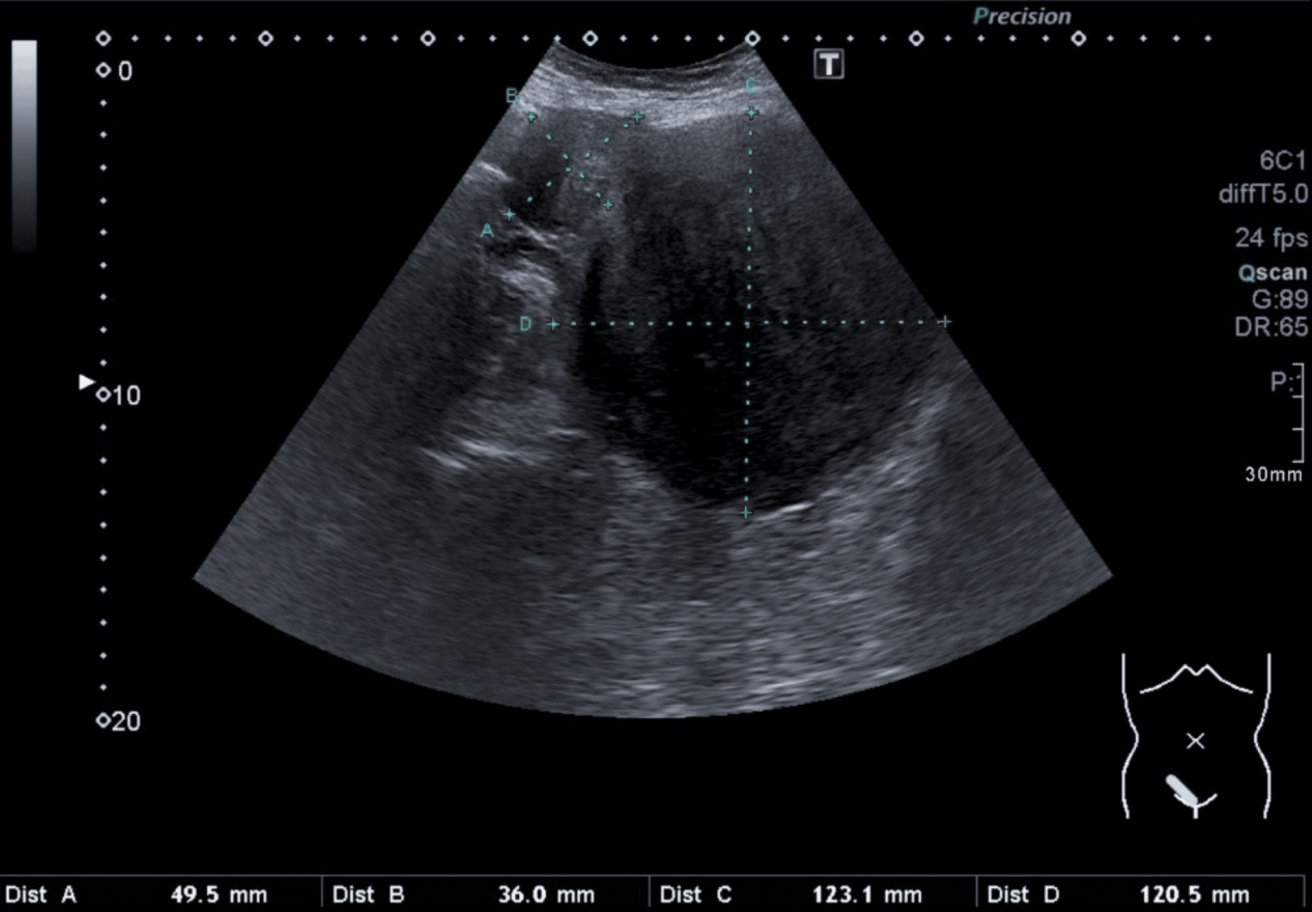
An abdominal ultrasound of the big tumorous mass in the uterus and the smaller mass next to it
